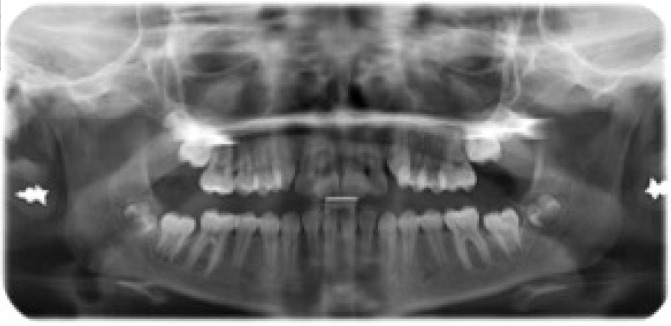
Initial panoramic x-ray.
